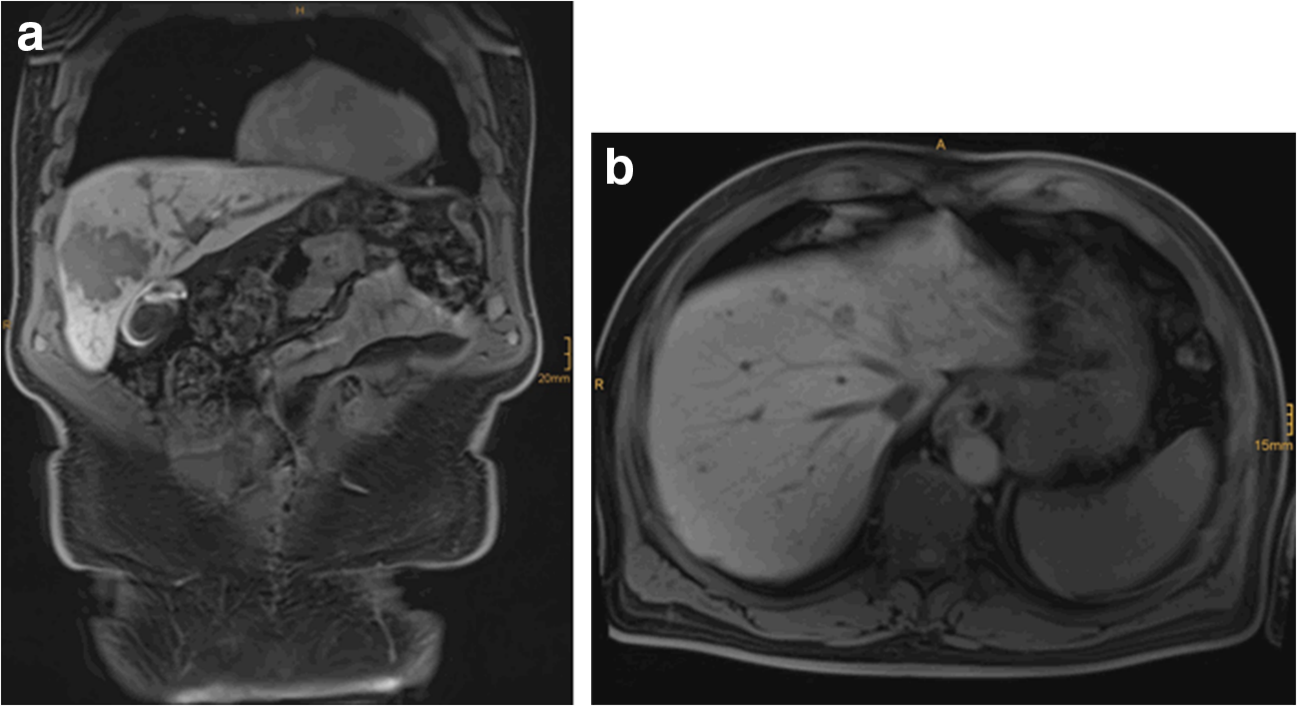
Bilobar CRLM; preoperative contrast-enhanced MRI of the liver, liver specific phase (12.04.2019). a coronal plane, b transverse plane
